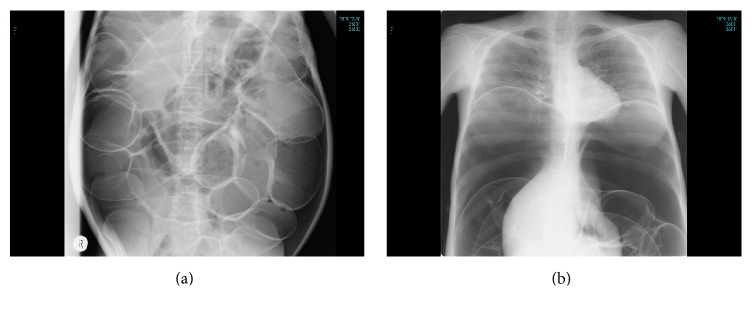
Plain radiographs ((a) lateral view of the abdomen and (b) frontal view of the chest and abdomen) show extensive gas in the colon wall, a dominance of air in the abdominal cavity above the colon, and secondary diaphragmatic elevation.
